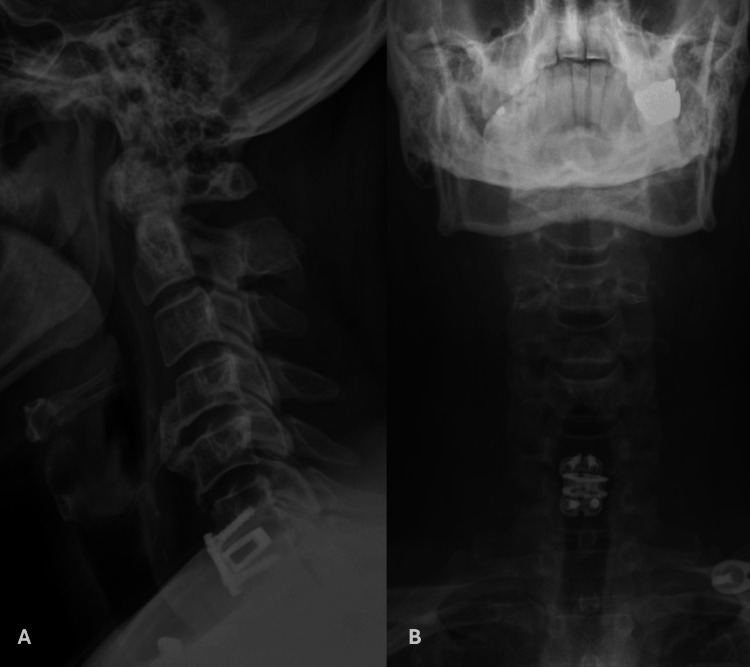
Postoperative X-rays showing appropriate alignment of the interbody spacer along with cervical plate and screws​ Pane A: Lateral cervical spine X-ray. Pane B: Anterior-posterior cervical spine X-ray.
